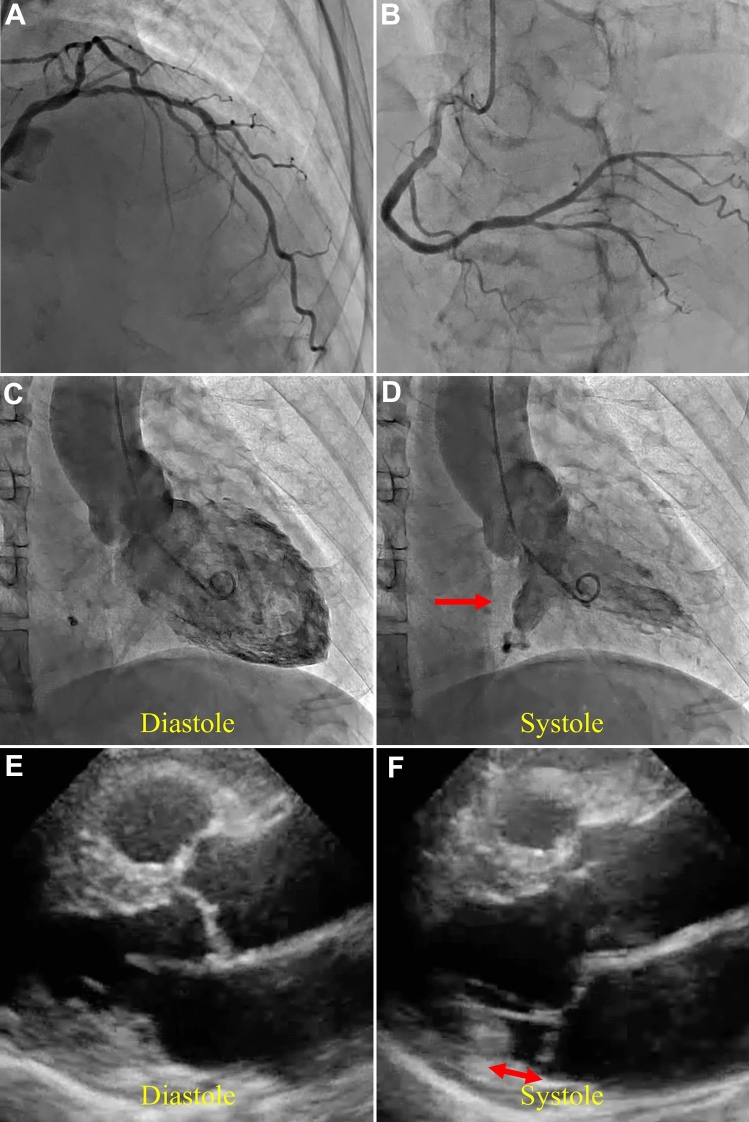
A Initial coronary angiogram (CAG) demonstrating significant stenosis in the proximal left anterior descending artery (LAD). B CAG demonstrating no significant stenosis in the right coronary artery. C Left ventriculography (LVG) during diastole. D LVG during systole. (red arrow: aneurysm-like structure). E Transthoracic echocardiography in long-axis view during systole. F Transthoracic echocardiography in long-axis view during diastole
